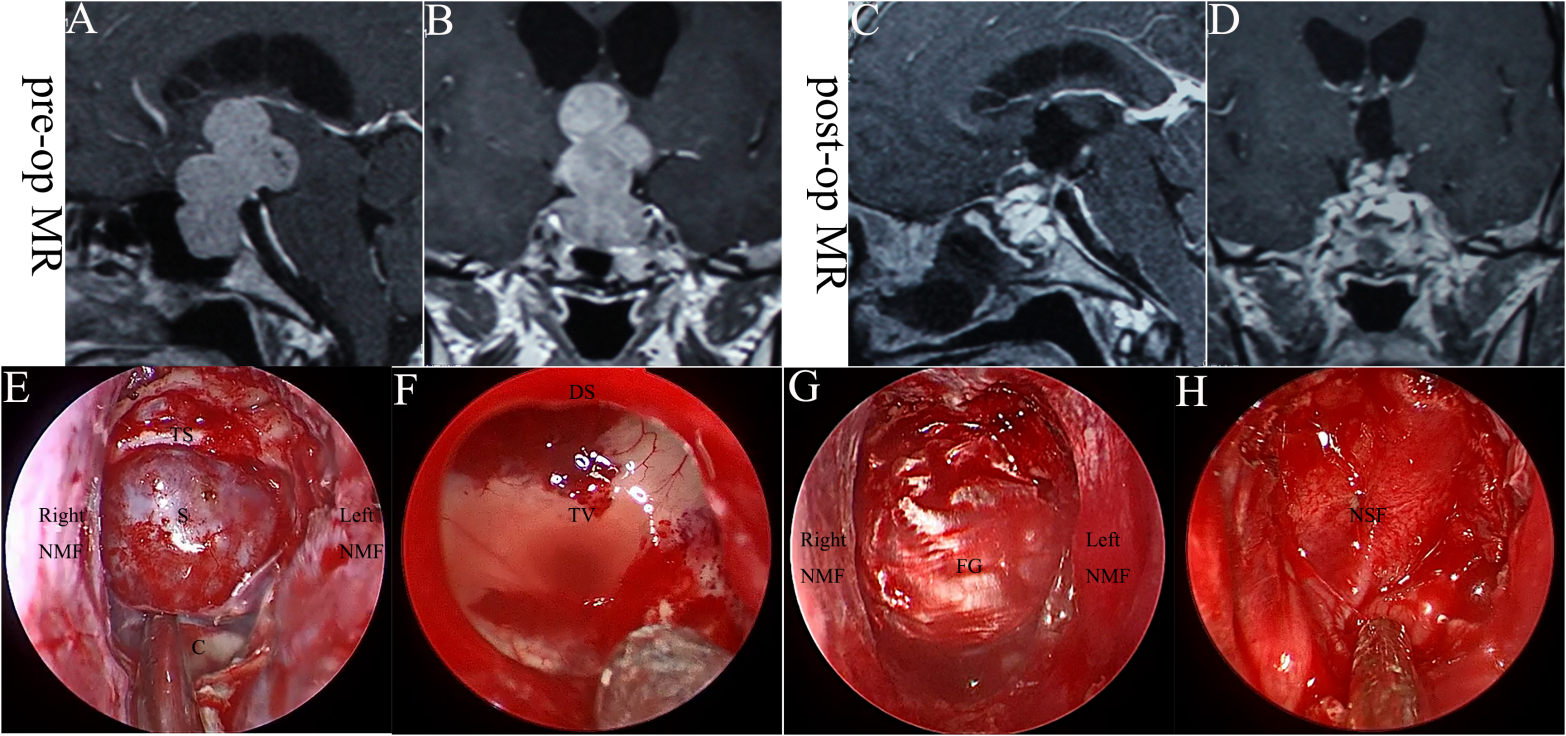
The case with high-flow CSF leak was reconstructed with a nasoseptal flap. (A, B). Pre-operative MRI scanning. (C, D). Post-operative MRI scanning. (E–H). Intraoperative images. S, sella; C, clivus; TS, tuberculum sellae; NMF, nasoseptal mucosal flap; DS, diaphragma sellae; TV, the third ventricle; FG, fascial graft; NSF, nasoseptal flap.
